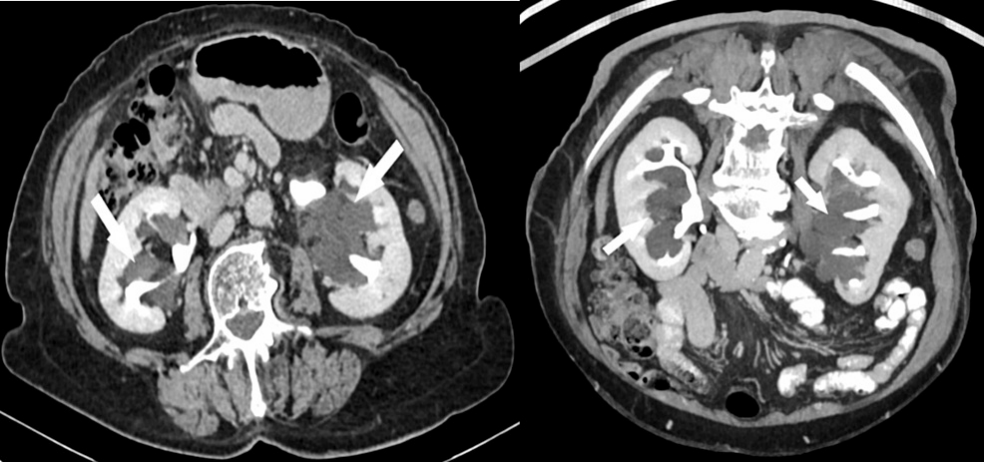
The axial and oblique coronal contrast-enhanced CT (CECT) images captured during the excretory phase reveal multiple cysts, bilaterally distributed and originating within the kidney parenchyma, which are prominently displayed. These parapelvic cysts extend into the renal sinus region, indicating their involvement with the anatomical structures surrounding the kidneys. This figure is the original work of the authors. Patient consent for the use of the image was obtained, as mentioned in the patient consent form (point no. 12).
